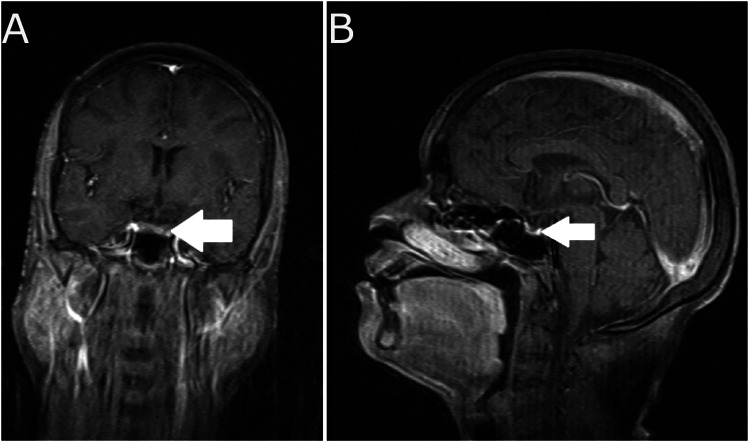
(A) T1 weighted MRI of the coronal section of the brain; (B) T1 weighted MRI of the sagittal section of the brain The scans reveal thinning of the pituitary gland with partially empty sella (arrows). However, the pituitary stalk is normal in length and width. MRI: magnetic resonance imaging
